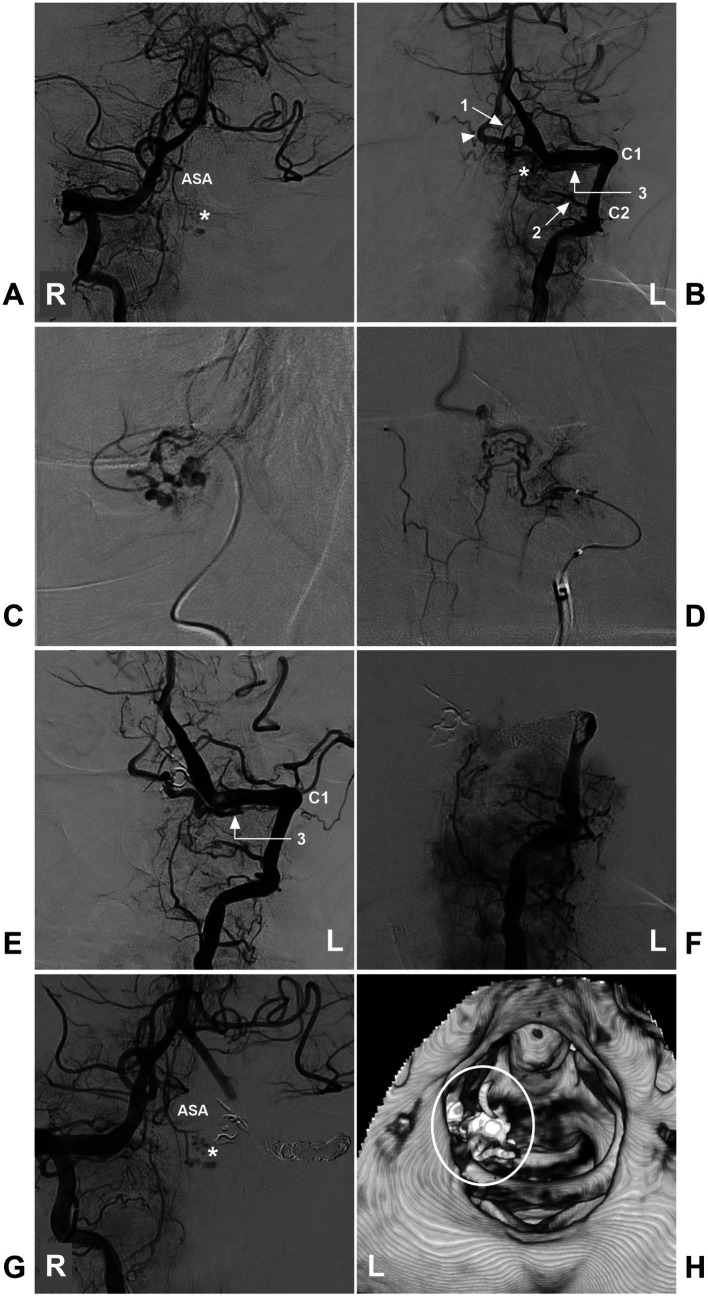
Incomplete EVT in a high cervical CCJ RAVF. (A) DSA of the left VA showing that the ASA supplied the AVF (asterisk). (B) DSA of the right VA showing that the AVF (asterisk) was fed by a brainstem perforating branch of the VA (arrow with number 1), the C2 (arrow with number 2) and C1 (arrow with number 3) radicular arteries, and the arrowhead indicates the draining vein. (C) Selective angiography of the brainstem perforating branch of the VA (arrow with number 1 in B) showing it to supply a part of the AVF. (D) Selective angiography of the C2 radicular artery (arrow with number 2) showing it to supply a part of the AVF. (E) After casting Onyx via two feeding arteries (numbers 1 and 2 in B), DSA of the left VA showing that the AVF was supplied by the C1 radicular artery (number 3 with arrow), confirming RAVF presentation. (F) DSA of the left VA showing that the VA was completely occluded by coiling, and the AVF could not be seen. (G) DSA of the right VA showing a part of the AVF (asterisk) was left. (H) Post-EVT CT reconstruction showing casting Onyx (ellipse), indicating the location of the RAVF. ASA, anterior spinal artery; AVF, arteriovenous fistula; C1-2, first and second cervical vertebrae; CCJ, craniocervical junction; CT, computed tomography; DSA, digital subtracted angiography; EVT, endovascular treatment; L, left; R, right; RAVF, radicular AVF; VA, vertebral artery.
